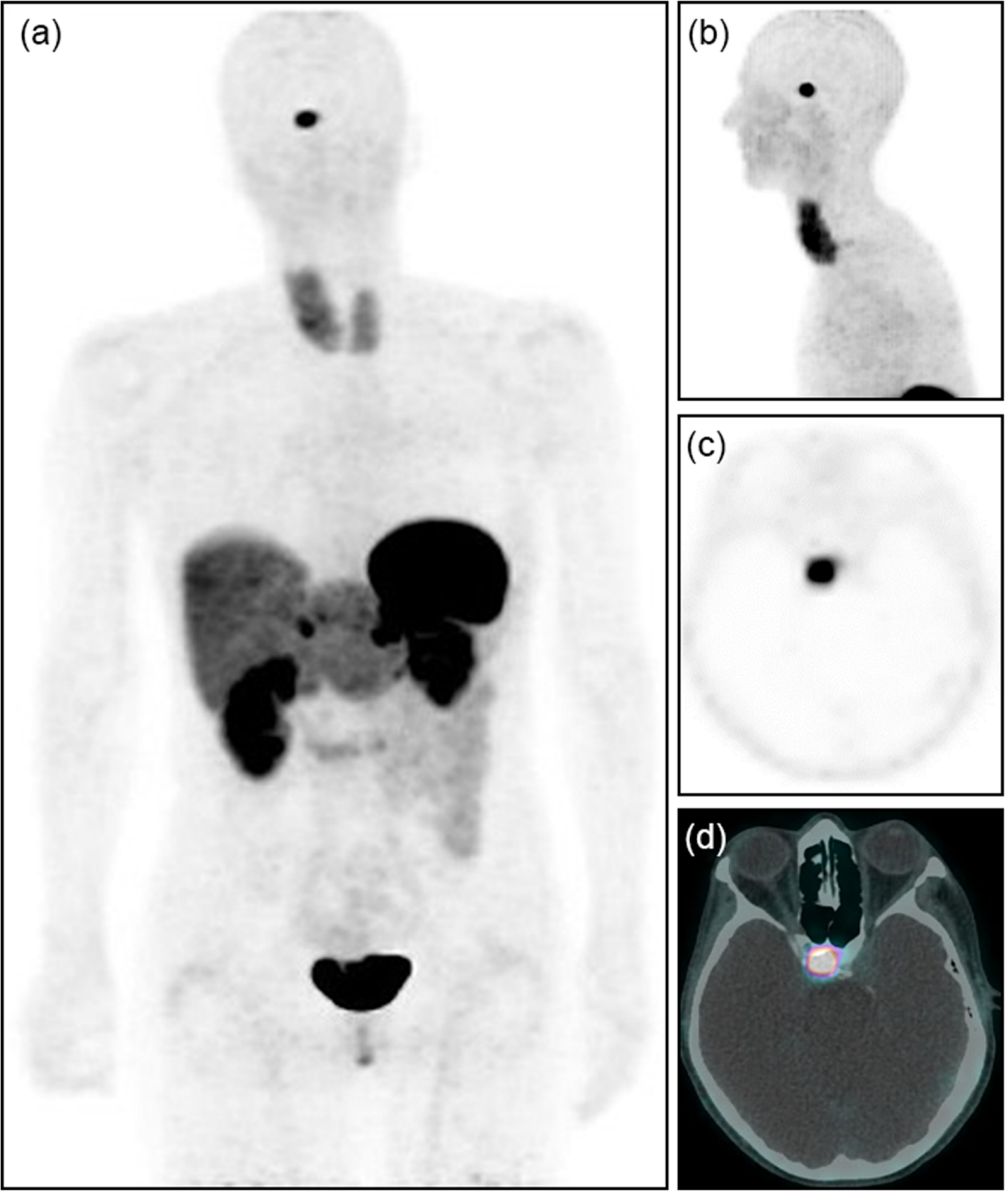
A 50-year-old woman was referred with recurrent central hyperthyroidism, 2 year post pituitary surgery for a TSH secreting micro-adenoma. [68Ga]Ga-DOTA-TATE PET MIP (a, b), trans-axial PET, and fused PET/CT images (c, d) clearly depict increased focal uptake (SUVmax 14.3) in pituitary fossa. The patient underwent repeat surgical resection of recurrent pituitary adenoma (Thyrotropinoma)
